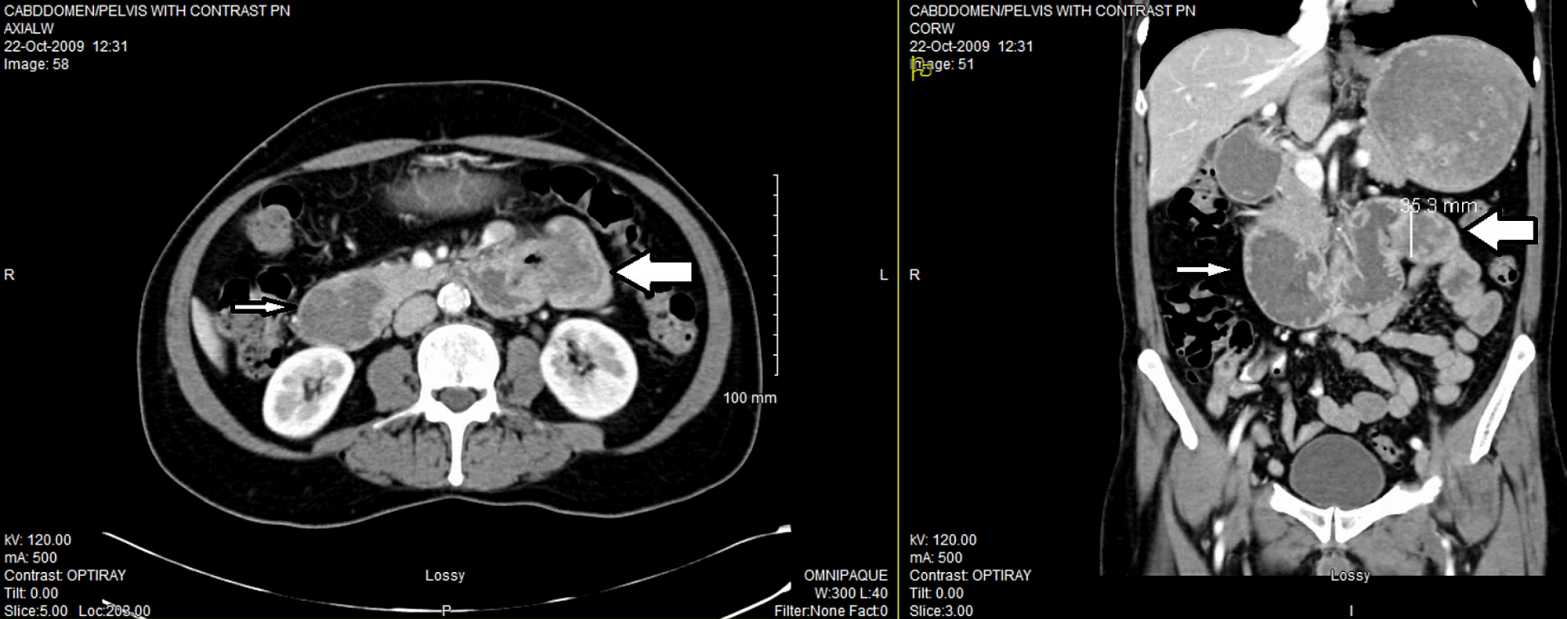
CT scan of the abdomen and pelvis in October 2009 showing a polypoid mass of the proximal jejunum (big arrow), incomplete bowel obstruction, marked dilatation of the stomach, and the duodenum (small arrow).
